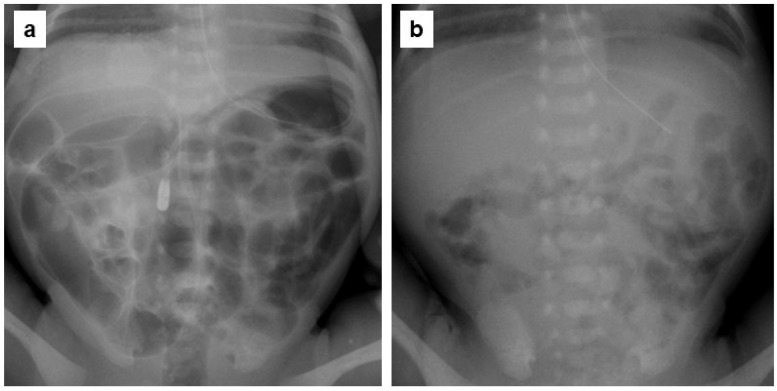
Plain abdominal radiographs of a very-low-birth-weight infant showing a marked daikenchuto (DKT) effect in the same patient. (a) Seven days after birth (2 days prior to DKT administration). The gas volume score (GVS) was calculated as 0.554. (b) Twelve days after birth (3 days after the start of DKT therapy). The GVS was calculated as 0.184.
